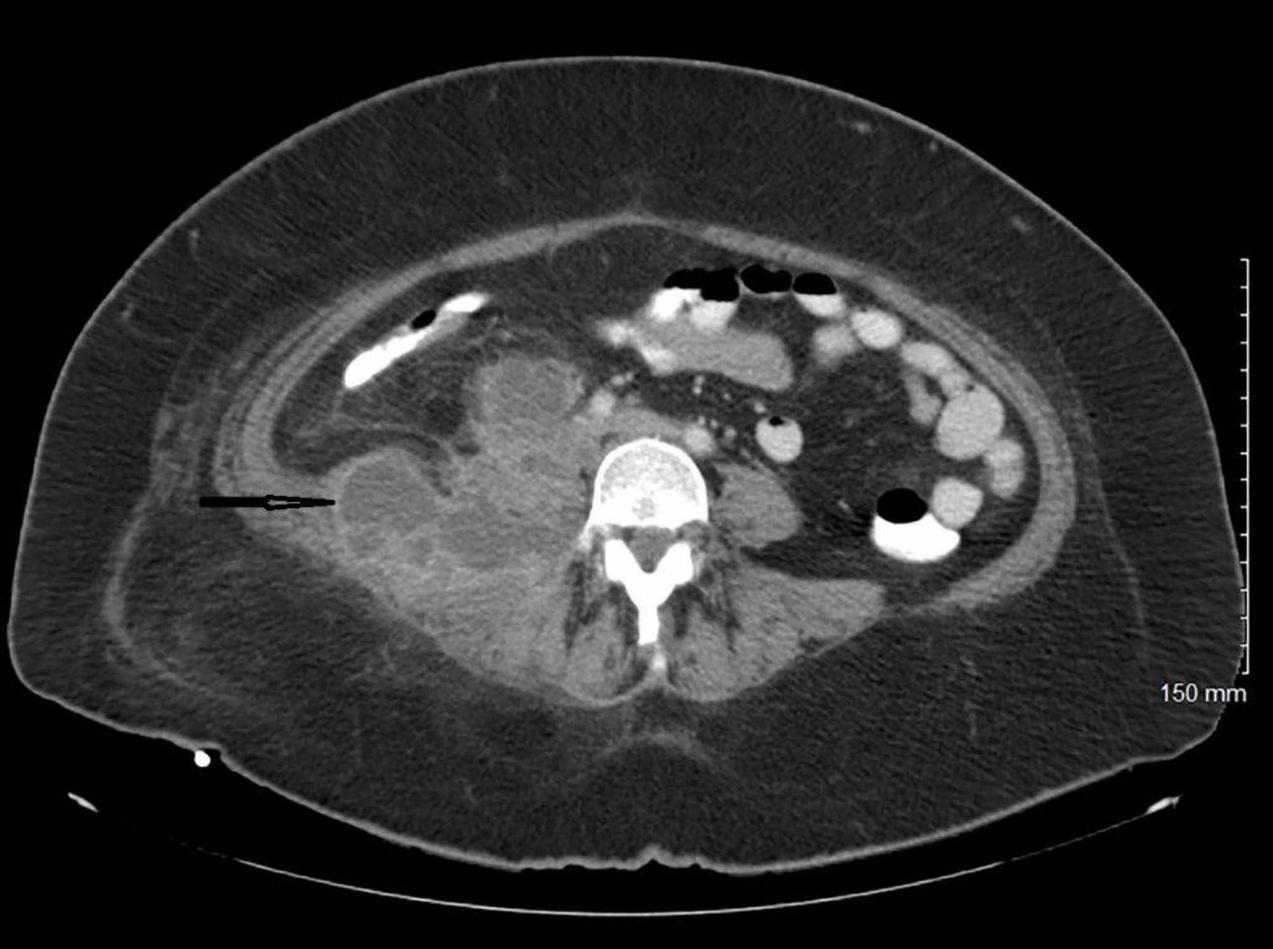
CT abdomen and pelvis with contrast after drainage and two days of antifungals Abscess in the right psoas and paravertebral muscles measuring 8 x 9 cm x 8 cm extending posterolaterally to involve the right external and internal oblique muscles CT: computed tomography
